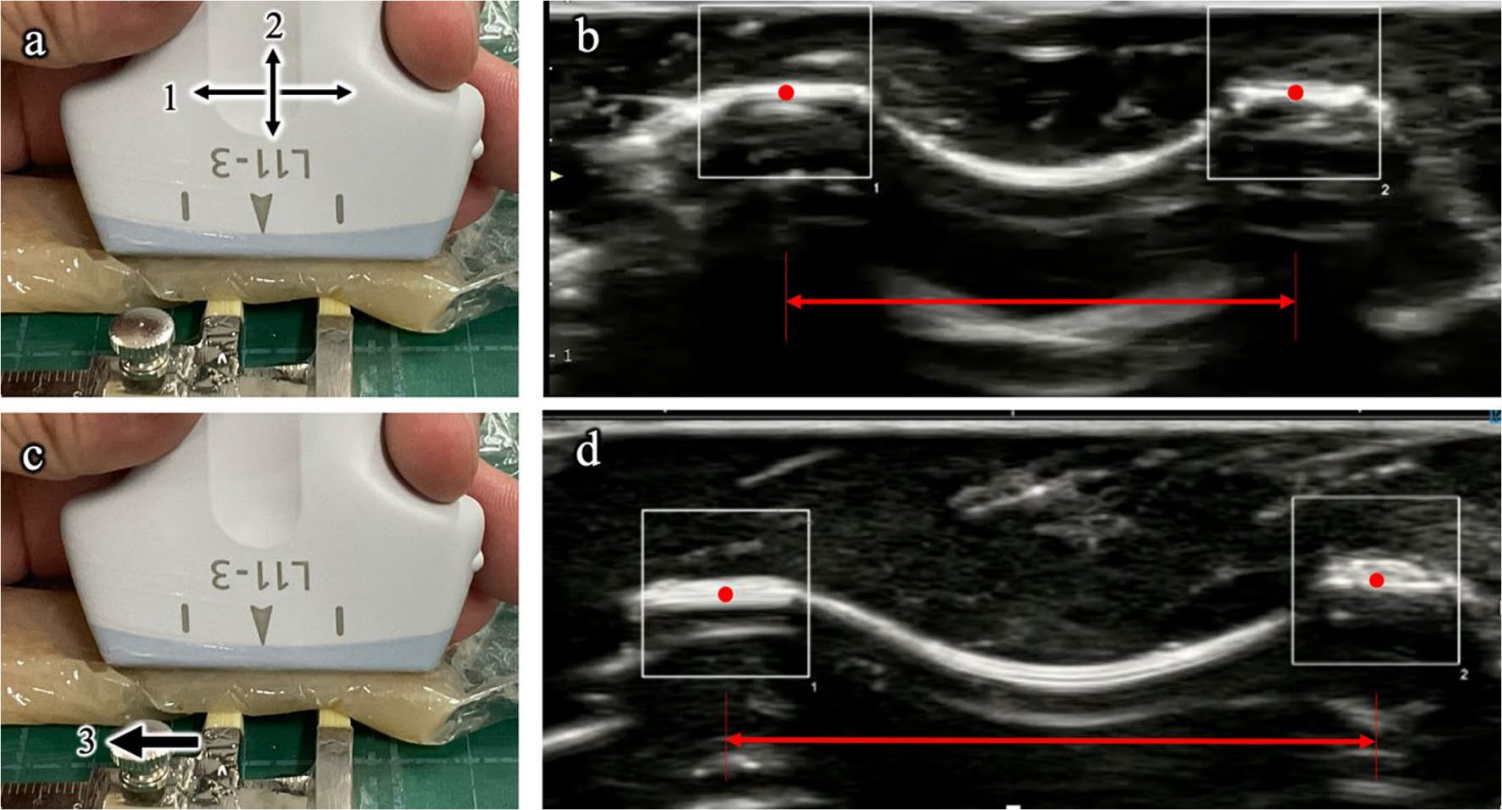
Setup of the reliability examination and ultrasound imaging. (a) Lateral (Task 1) and near-far (Task 2) probe movements along the 10-mm caliper distance using a chicken fillet as an intervening substance. (b) The red double arrow is the distance between two points of the region of interest (ROI) (small, white circles). (c) The caliper is expanded by 5 mm to 15 mm and then manually returned to 10 mm (Task 3). (d) The red double arrow is the distance between two points of the ROI, tracking the expansion of the caliper to 15 mm.
